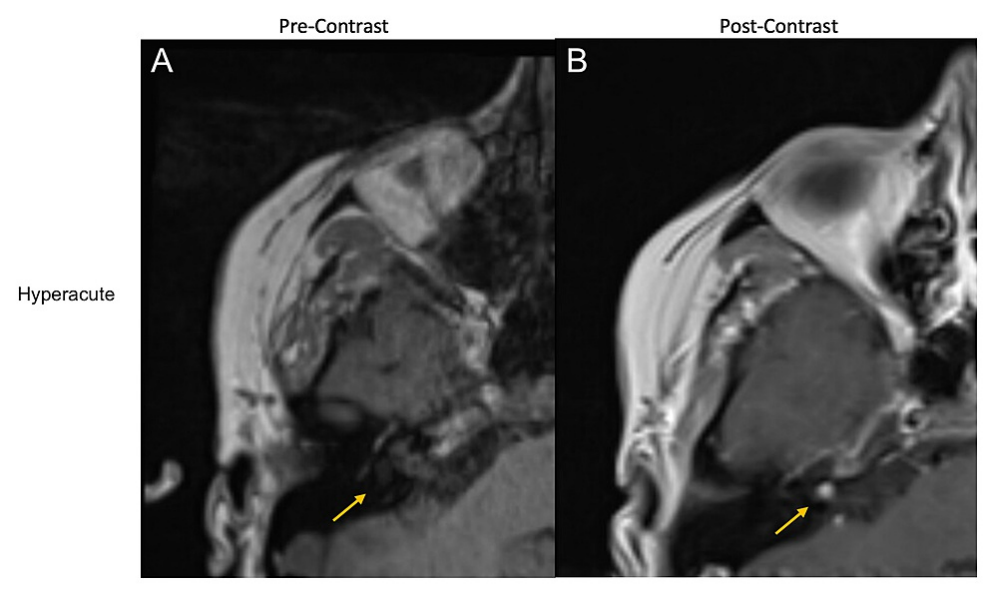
Right IAC lesion on MRI with and without contrast at hyperacute (time of presentation) time point A: Pre-gadolinium T1-weighted axial MRI brain demonstrating the right IAC during hyperacute presentation with the isointense appearance of the lesion B: Post-gadolinium T1-weighted axial MRI brain showing a rounded 4-mm homogenously enhancing lesion in the right IAC (hyperacute) IAC: internal auditory canal; MRI: magnetic resonance imaging
